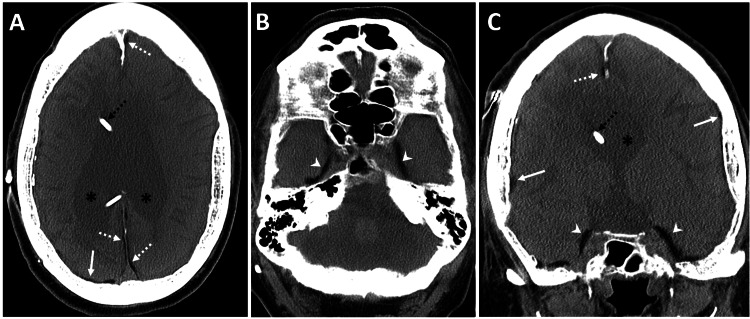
Diffuse dural fat deposition and cerebral atrophy in a patient with chronic VP shunting. A 66-year-old male with a medical history of altered mental status, in the context of known severe mental retardation, cerebral palsy, and a VP shunt. Unenhanced CT images of the brain, obtained with soft tissue settings - (A and B) axial and (C) reformatted coronal views - show diffuse atrophy of the brain parenchyma related to known cerebral palsy, with prominent bilateral lateral ventricles (black asterisks in A and C) and a right frontal approach VP shunt catheter (dashed black arrow in A and C). Incidentally, a diffuse dural fat collection (Hounsfield Unit -70) is seen along the falx cerebri (dashed white arrows in A and C), cerebral convexities (solid white arrows in A and C), and middle cranial fossa (white arrowheads in B and C), without sulcal or basal cistern fat densities. There is associated calvarial hyperostosis from chronic venous shunting. VP, ventriculoperitoneal; CT, computed tomography
